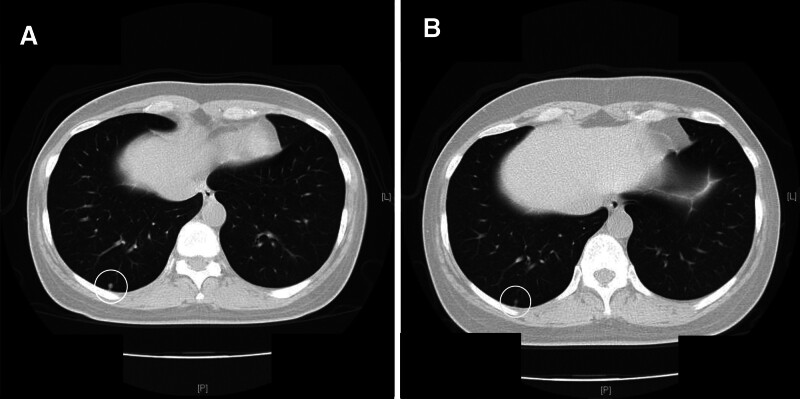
LDCT image of a case taken for 1-yr period. (A) LDCT showing a small nodule located in the right lower lung field with a slight pleural indentation (circled) at first examination. (B) LDCT of this case 1 yr after the first examination. The nodular shadow shows rather improvement (circled) compared with that at the first examination. LDCT = low-dose computed tomography.
